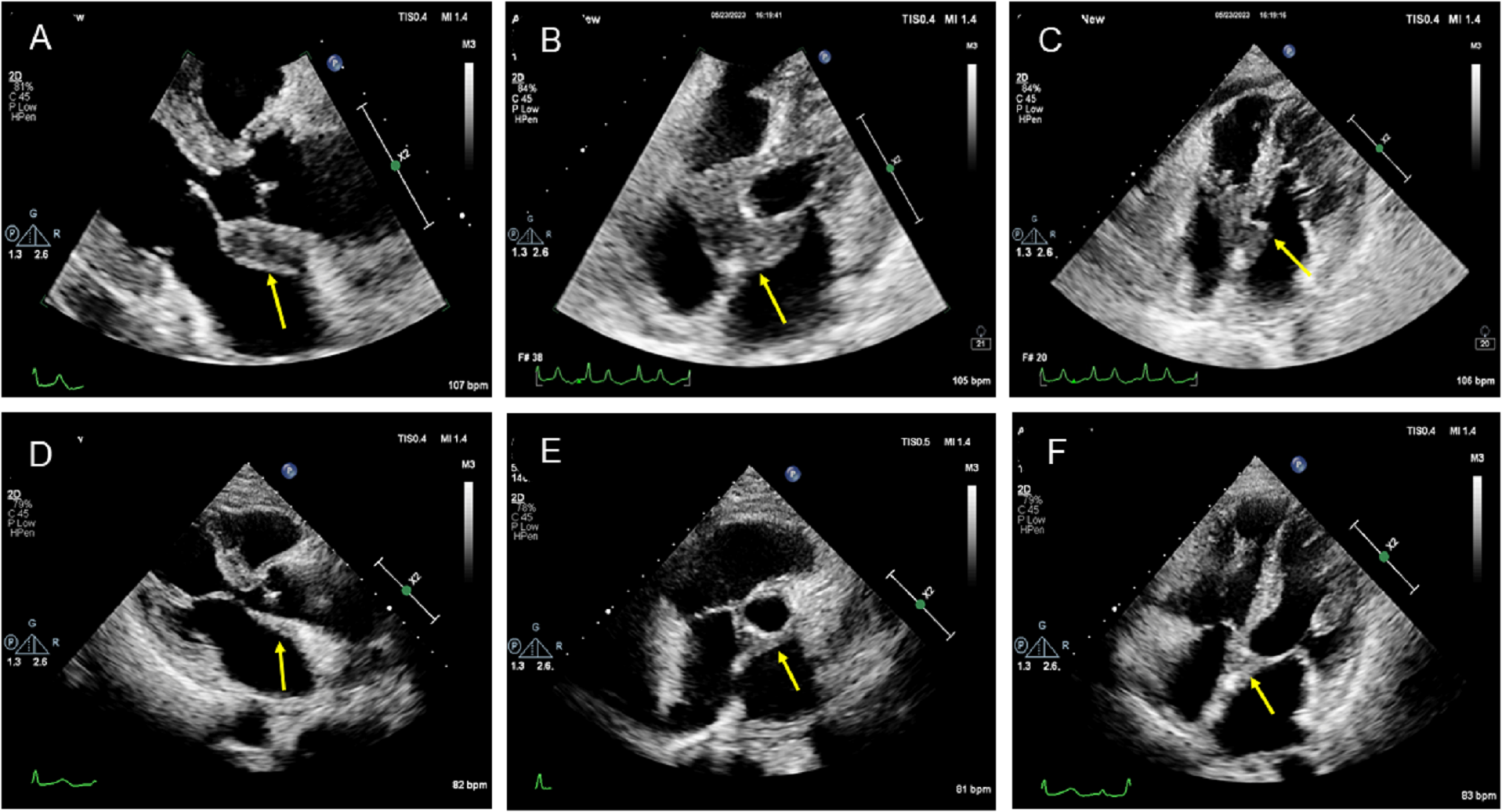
Two-dimensional transthoracic echocardiographic manifestation of the lesion in the aortic root. (A–C) Two-dimensional transthoracic echocardiography revealed an isoechoic mass around the aortic root with a clear boundary, irregular shape, uneven internal echo, and no calcification. (D–F) Transthoracic echocardiography showed that the mass around the aortic root was smaller after treatment.
